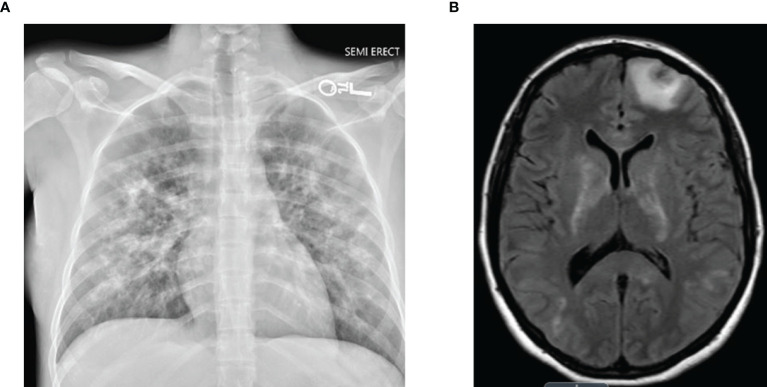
(A) Chest X-ray revealing diffuse interstitial and airspace opacities. (B) Brain MRI revealing extensive fluid-attenuated inversion recovery (FLAIR) signal abnormalities throughout supratentorial parenchyma.
